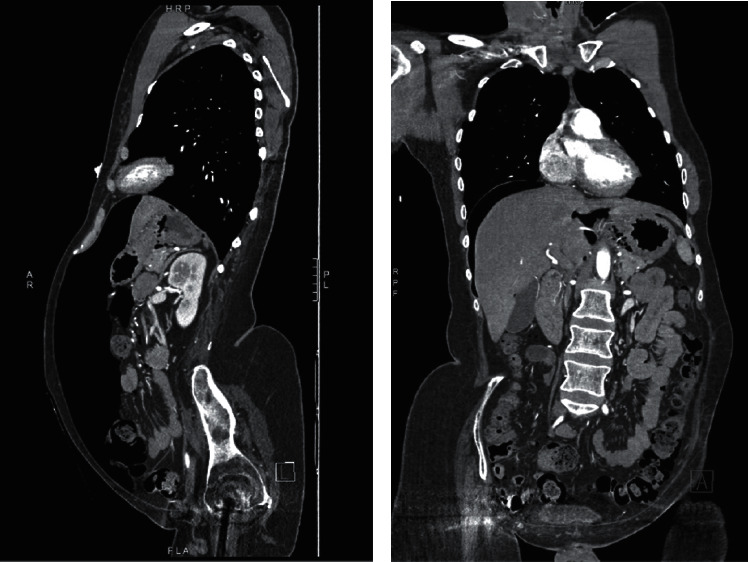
Computed tomography (CT) scan of the chest, abdomen, and pelvis; sagittal view (a) and coronal view (b).
